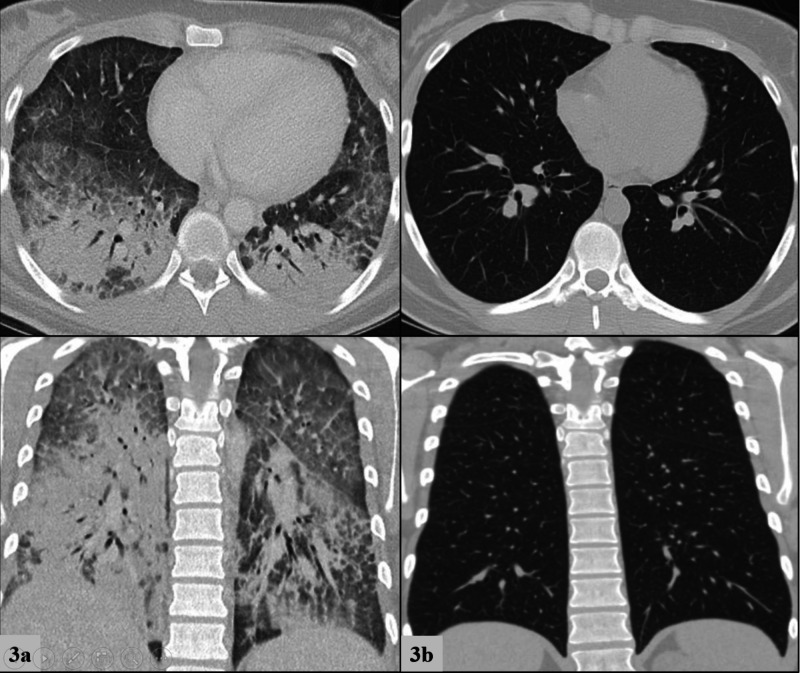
Chest computed tomography for Patient 3 at presentation (3a, left column) compared to 1 month after discharge (3b right column). Top row frames depict transverse views and bottom row frames depict coronal views.
